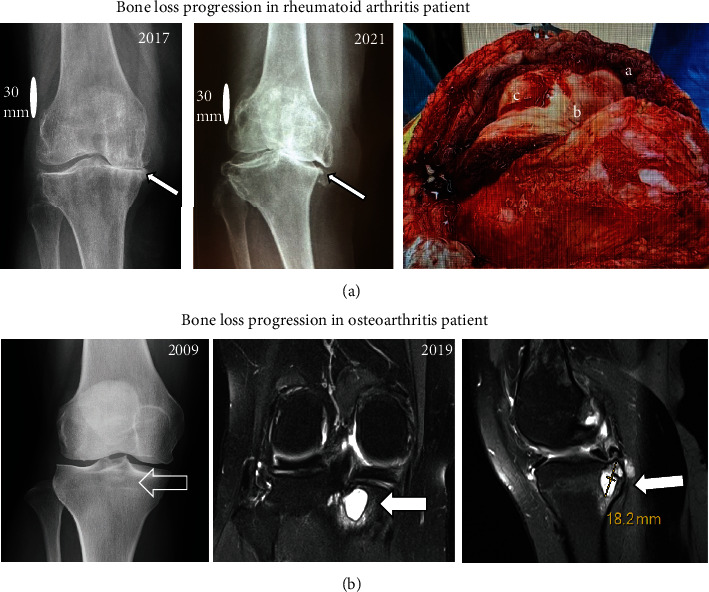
Clinical changes seen in RA and OA patient knees. Panel (a) shows the progression of erosive arthritis due to RA in a knee of female patient during 2017 to 2021 and intraoperative picture during a knee prosthesis operation demonstrates synovial inflammation and hypertrophy (a) along with cartilage (b) and bone damage (c) associated with synovial hypervascularity and oedema. The medial tibial plateau (arrows) is heavily eroded during the follow-up time period. Panel (b) shows X-ray and MRI images of a young female's knees. At the age of 27, only some early OA changes are seen. The magnetic resonance images ten years later show progression of OA, with large subchondral bone loss and a cyst in the proximal tibia as an indication of increased bone degradation.
